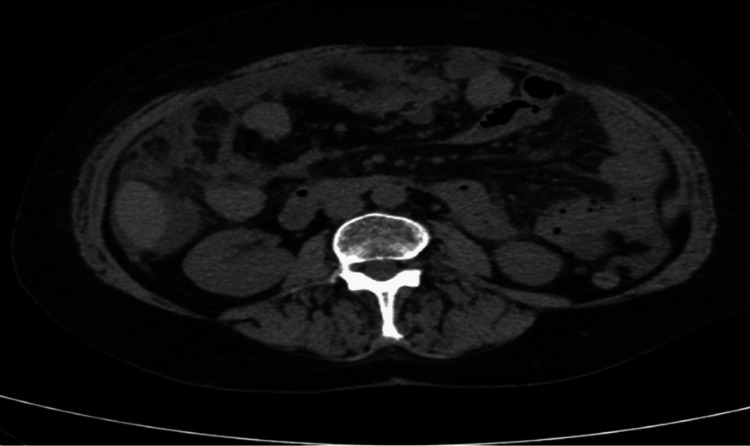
Computed tomography of the abdomen revealed densification of the omentum, suggesting peritoneal carcinomatosis and secondary involvement.
